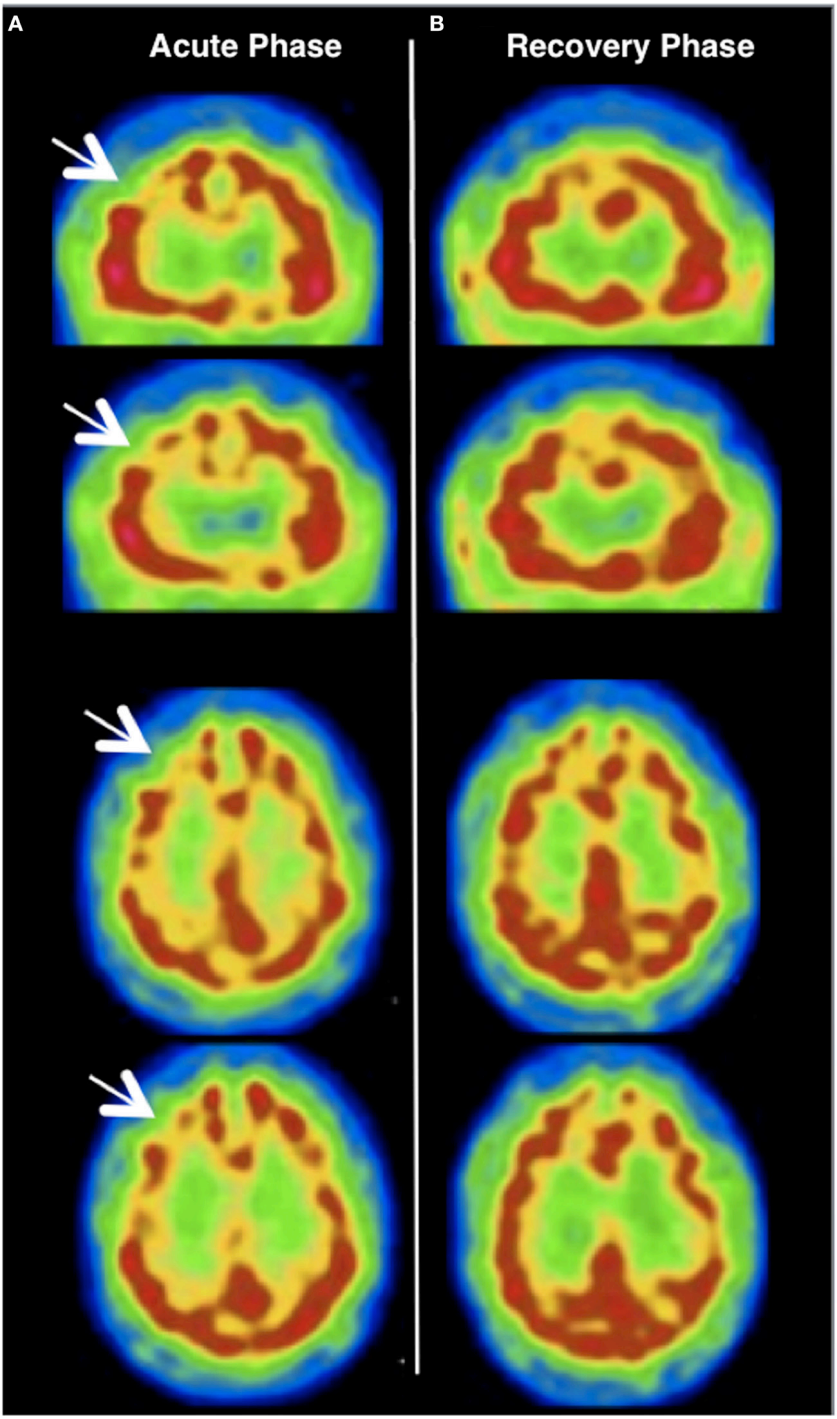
(A) Brain 99mTc-hexamethylpropyleneamine oxime single-photon emission computerized tomography (99mTc-HMPAO SPECT) performed 13 days after the onset of symptoms revealing a significant decrease in regional cerebral blood flow (rCBF) within the right dorsolateral prefrontal cortex (see white arrows); (B) a 10-month control 99mTc-HMPAO SPECT showing a complete cancelation of the right frontal rCBF decrease present during the symptoms.
